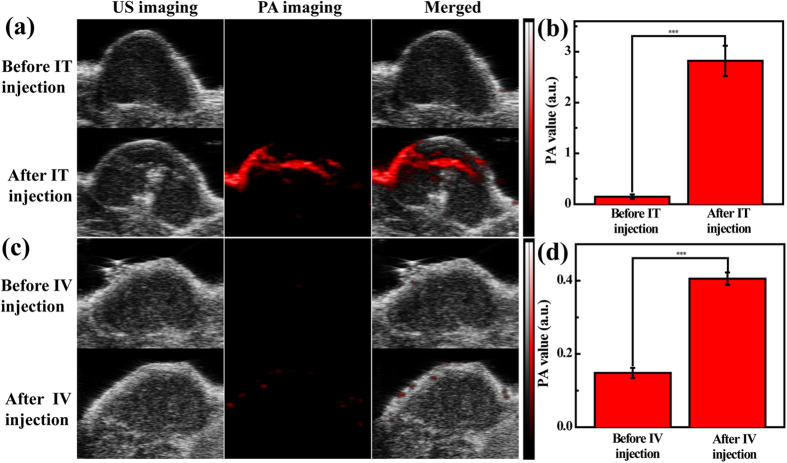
In vivo* PA imaging of a xenografted tumor model. *In vivo* ultrasound/PA images (a,c) and PA value (b,d) of tumors before and at 0.5 h post IT ([Au] = 100 mM, 0.04 mL in PBS, a,b) and at 6 h post IV ([Au] = 100 mM, 0.1 mL in PBS, c,d) injection of the Fe_3_O_4_ @ Au-PEI.Ac-PEG-FA NSs.
